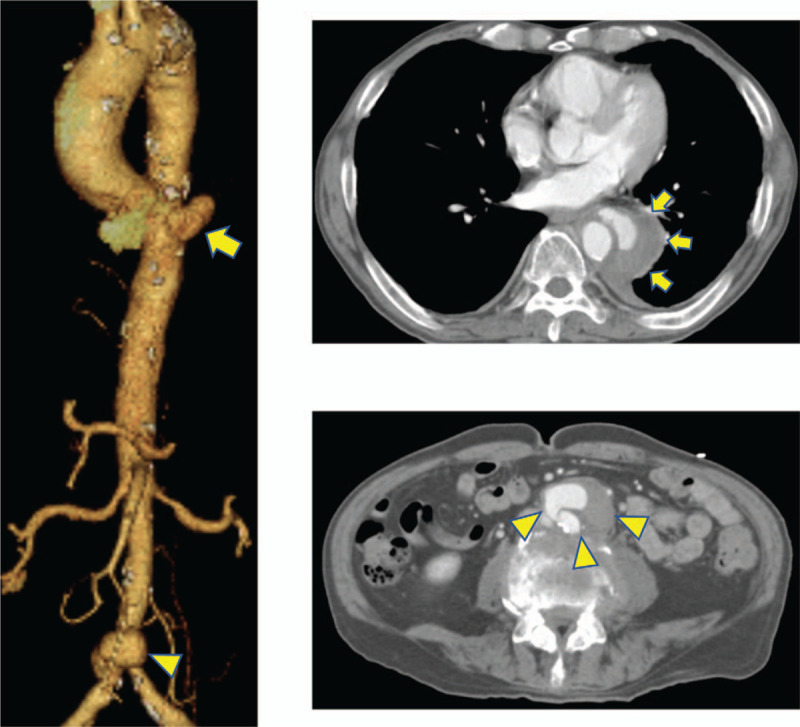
Preoperative CT. Follow-up CT at 4 weeks after the initial examination showed enlargement in size of both aneurysms (TAA, 39 × 35 mm–52 × 47 mm [arrow], AAA, 26 × 23 mm–41 × 34 mm [arrowhead]). The surrounding tissue showed typical findings of inflammation, such as thickening of the adventitia and faint contrast effect. AAA = abdominal aortic aneurysm, CT = computed tomography, TAA = thoracic aortic aneurysm.
